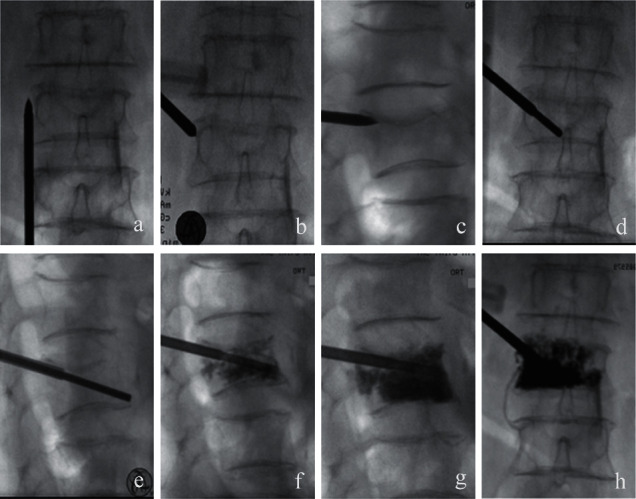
Anteroposterior and lateral views of the needle trajectory inserted into the vertebral body via a unilateral extrapedicular puncture method. (a) Anteroposterior radiograph showed the left transverse process of the fractured vertebral body. (b, c) The needle tip of the bone entry point was located at the bone groove at the junction between the pedicle and the vertebral transitional location. (d, e) Anteroposterior and lateral radiographs showed the optimal position of the working cannula. (f, g) The position of the cement cannula could be adjusted according to the dispersion of cement during the operation. (h) Anteroposterior radiograph showed the bone cement distribution.
